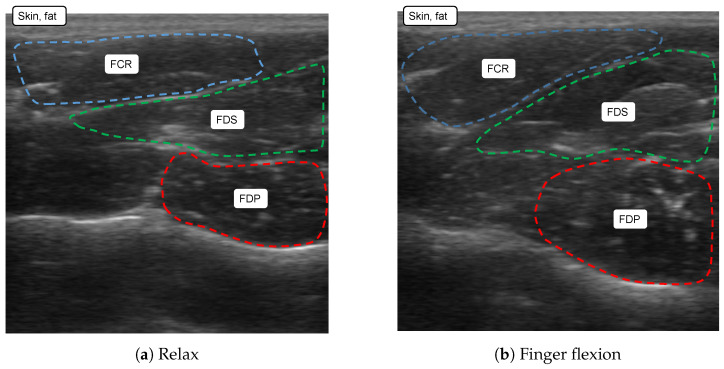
Forearm cross-section with ultrasound.
